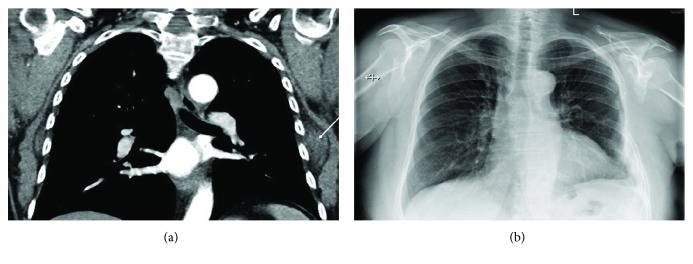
Chest radiography before and after the first operation. (a) Before the operation; arrow: the chest wall tumour. (b) At discharge.
